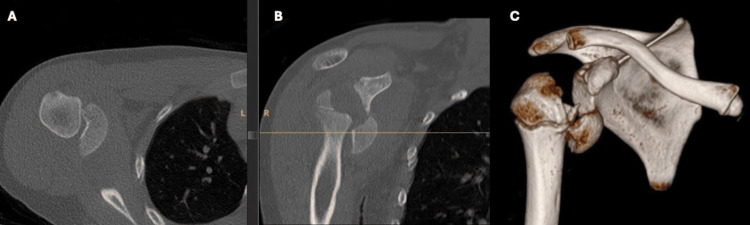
(A) Selected axial and (B) coronal computed tomography images of the SH III injury with (C) 3D reconstruction.
